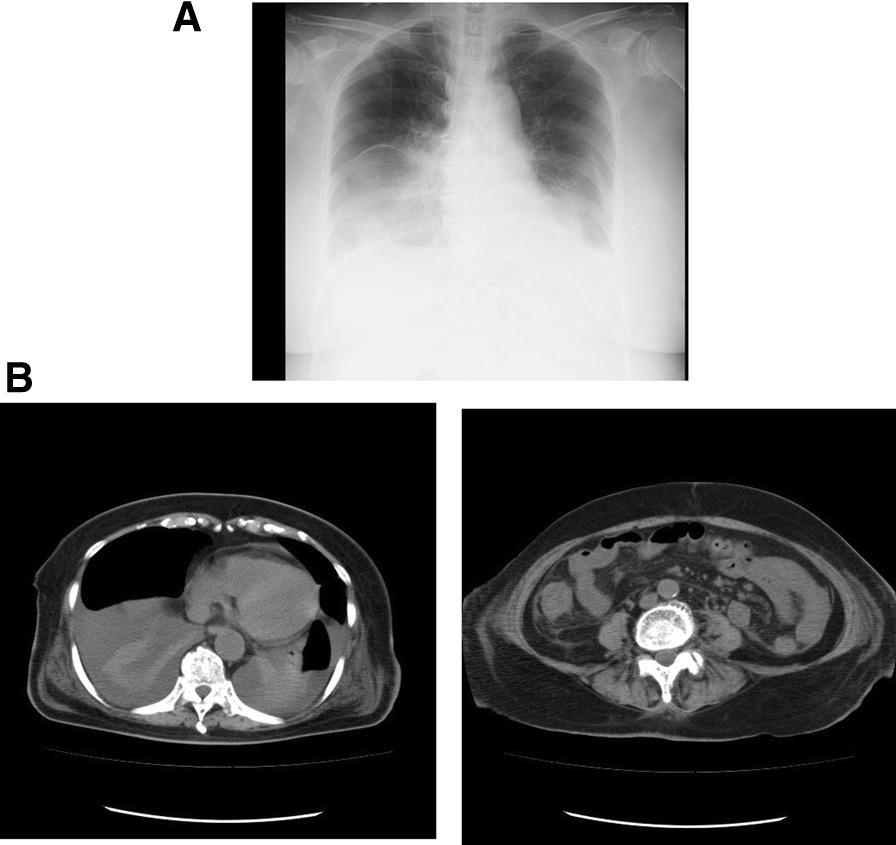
A Chest radiography. Chest radiography revealed massive pleural effusion. B Plain computed tomography (CT) upon transfer to our hospital. CT revealed massive pleural effusion, some ascites, and the thickening of the wall of the small intestine
